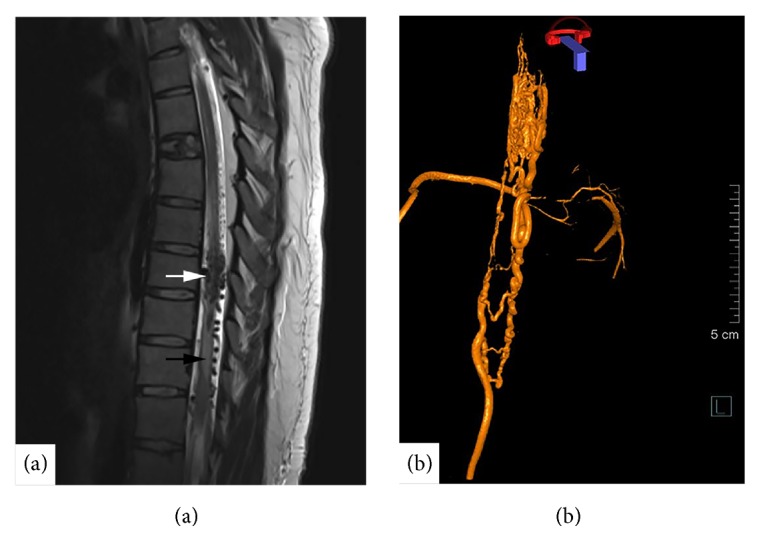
(a) Lateral T2-weighted MRI thoracic spine showing peri- and intramedullary spinal AVM at T8 (white arrow) with surrounding spinal cord oedema T6-T11 (black arrow). (b) Left lateral three-dimensional rotational spinal angiography (3D-RSA) from right T9 intercostal artery showing spinal cord AVM.
